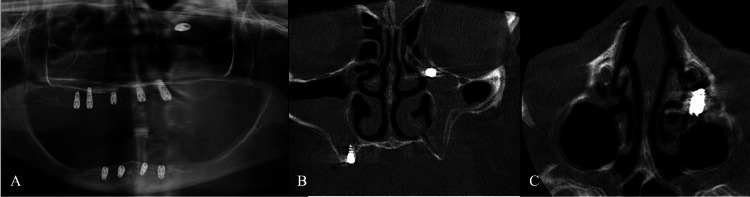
Radiological views of the displaced implant. Panoramic radiograph showing the displaced implant oriented horizontally and extending toward the orbital floor and nasal cavity (A). Coronal section from CBCT confirming proximity to the nasal cavity (B). Axial CBCT section showing horizontal orientation of the implant within the sinus (C) CBCT: cone-beam computed tomography
